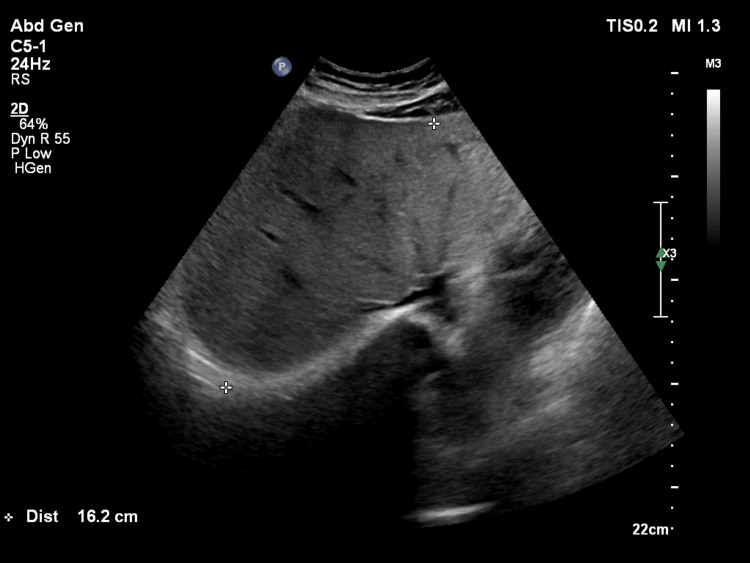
Ultrasound image of Grade II fatty liver
